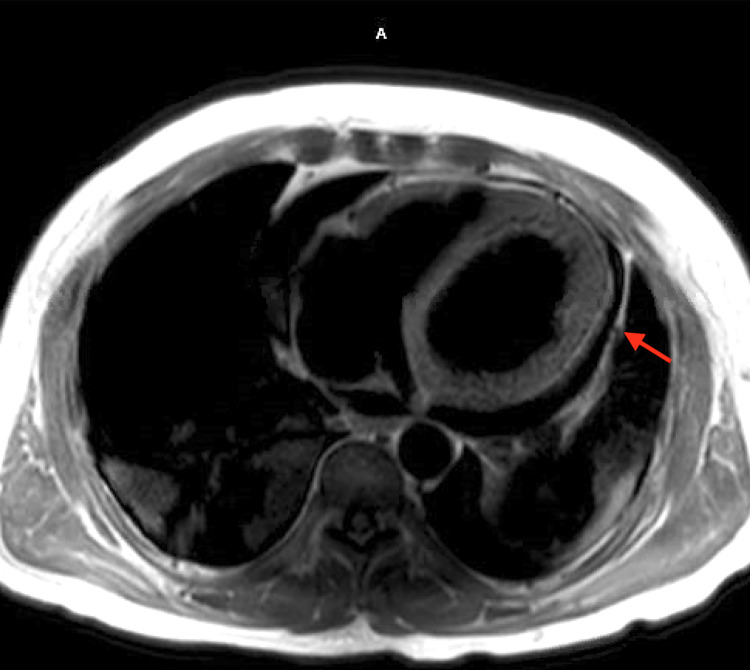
A cardiac magnetic resonance imaging short axis view showing a moderately sized pericardial effusion with slight myocardial enhancement in the inferior lateral wall (arrow).
